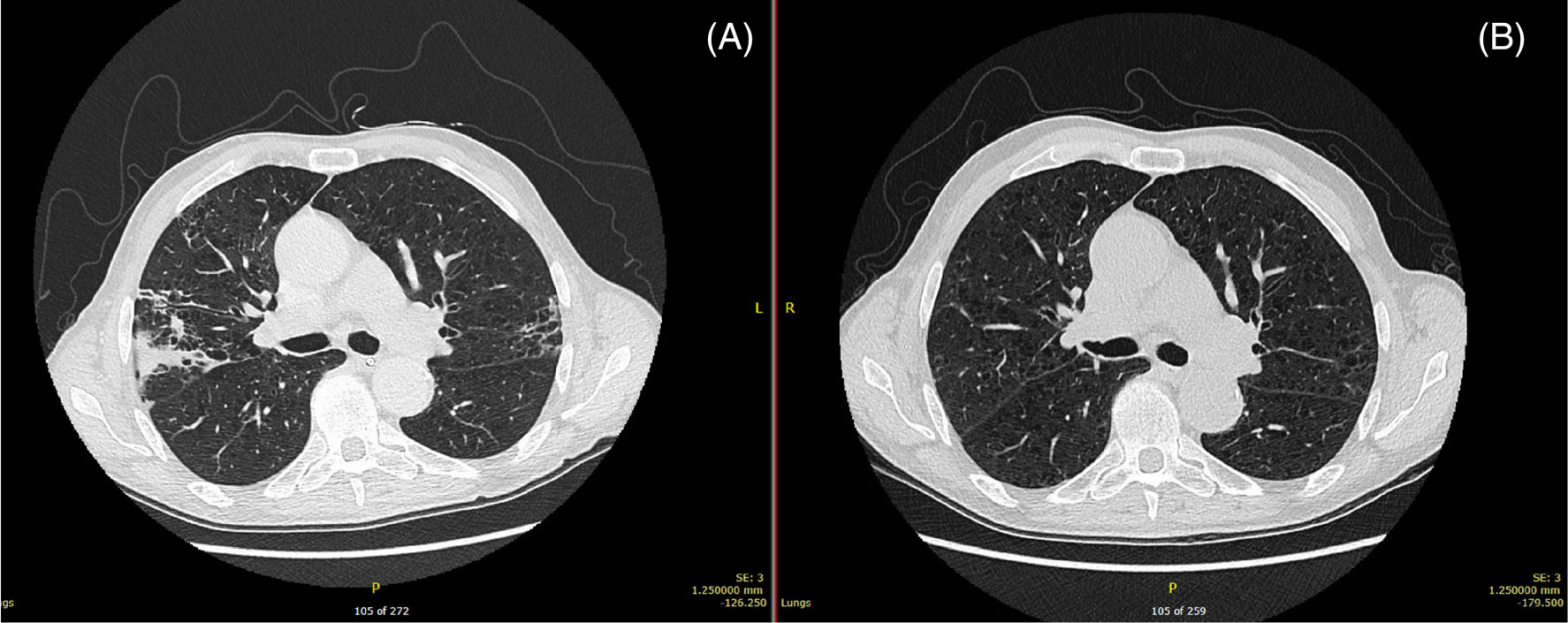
(A) Axial CT slice of multifocal pneumonia and (B) resolution
